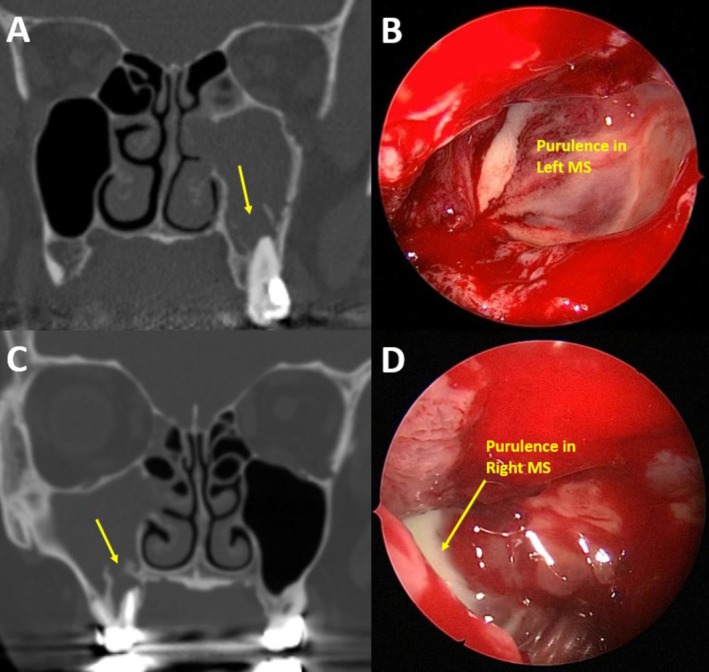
Two examples of odontogenic sinusitis (ODS) from apical periodontitis (A and B) and progressive periapical abscess following root canal treatment (C and D). (A) Coronal bone‐window CT showing left‐sided complete maxillary sinus opacification with an underlying molar with large periapical abscess with bone erosion (yellow arrow) in the setting of pulpal necrosis and apical periodontitis. (B) Left‐sided 70° endoscopic view of the left maxillary sinus (MS) following maxillary antrostomy, showing MS purulence. (C) CT showing right‐sided complete maxillary sinus opacification with an underlying molar with large periapical abscess with bone erosion (yellow arrow) following a prior root canal treatment. (D) 70° endoscopic view of the right maxillary sinus (MS) following maxillary antrostomy, showing MS purulence.
